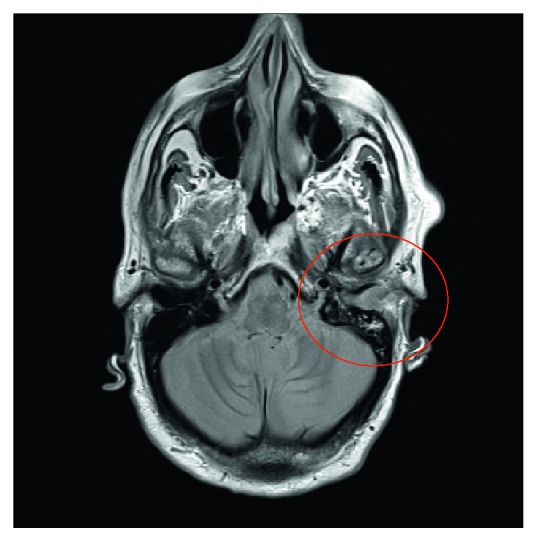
Brain MRI without and with contrast medium.
